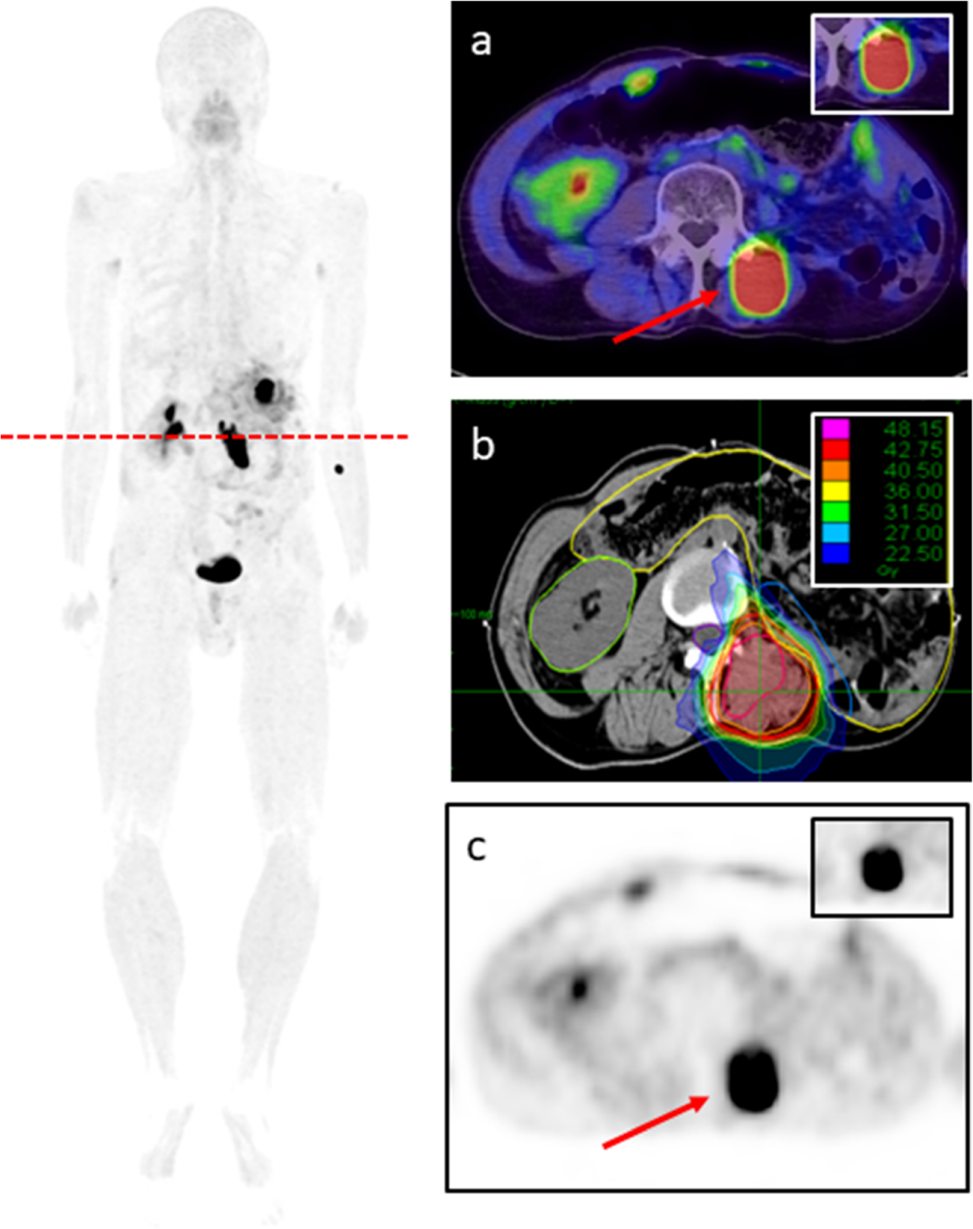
Local irradiation (b) of relapsed liposarcoma undergoing ^68^Ga-FAPI PET/CT (a, c). SUV_max_ 33.61 SUV_mean_ 18.27
